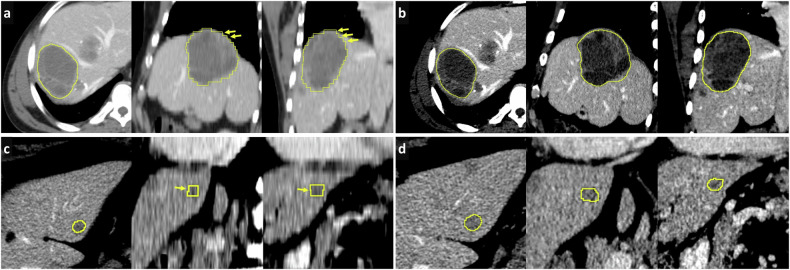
CT images showing liver metastasis segmentation performed on the axial plane in 5-mm slice thickness (a) vs 1-mm slice thickness (b), displayed in axial, sagittal, and coronal views. Thicker slices yield a volume of 175 cm³ compared to 167 cm³ for thinner slices—a 4.5% difference. The difference is even more noticeable in a smaller lesion, with volumes of 0.97 cm³ at 5 mm (c) compared to 0.85 cm³ at 1 mm—a 12.3% difference (d). Note the stair-step appearance (arrows) and reduced detail in the coronal and sagittal views for the thicker slices
